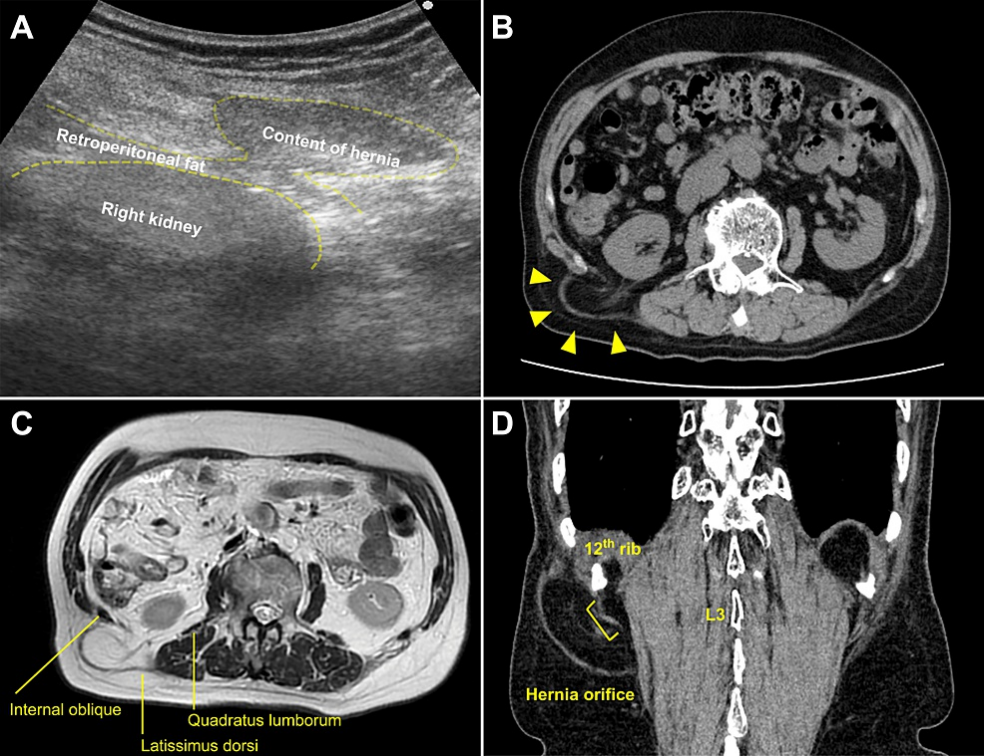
Preoperative imaging. A: Ultrasonography of the superior lumbar hernia. B: CT scan: Retroperitoneal fat directly protruding from the hernial orifice. C: MRI: The hernial orifice in relation to the 12th rib, internal oblique muscle, and quadratus lumborum muscle. D: The hernial orifice is oriented in relation to the right 12th rib and processus spinosus of the third lumbar vertebrae.
